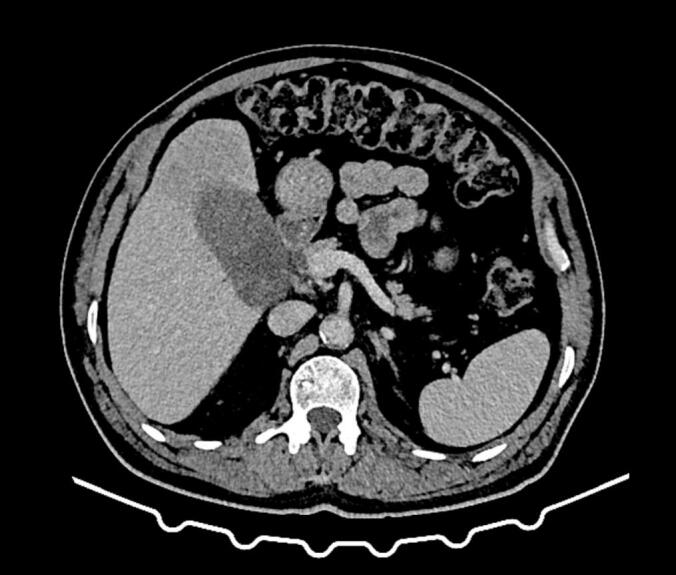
Contrast-enhanced axial CT scan demonstrating a mass involving the gallbladder fundus with contiguous extension into adjacent liver parenchyma (segment IV), suggestive of gallbladder malignancy.
